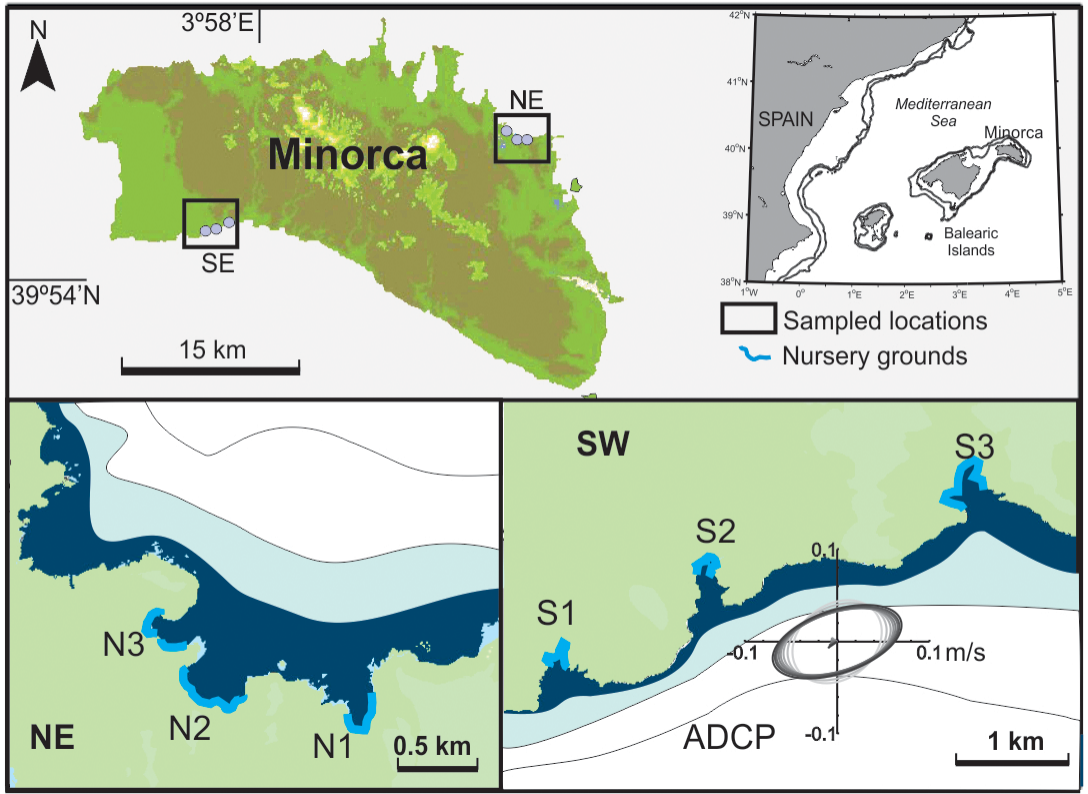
Study sites. The two distinct sampling locations at the Northeast (NE) and Southwest (SW) of the Menorca Island and the six coves monitored during the study. The Acoustic Doppler Current Profiler (ADCP) localization and the current variation ellipses measured at different depths (darker is shallower) are also indicated at the SW site. Maps were created by the authors by merging topographical maps generated with ArgGIS using IDEIB terrain elevation model (http://ideib.caib.es/visualitzador/visor.jsp) and the MATLAB software. There are no copyright restrictions associated and permissions for publication are not necessary.
